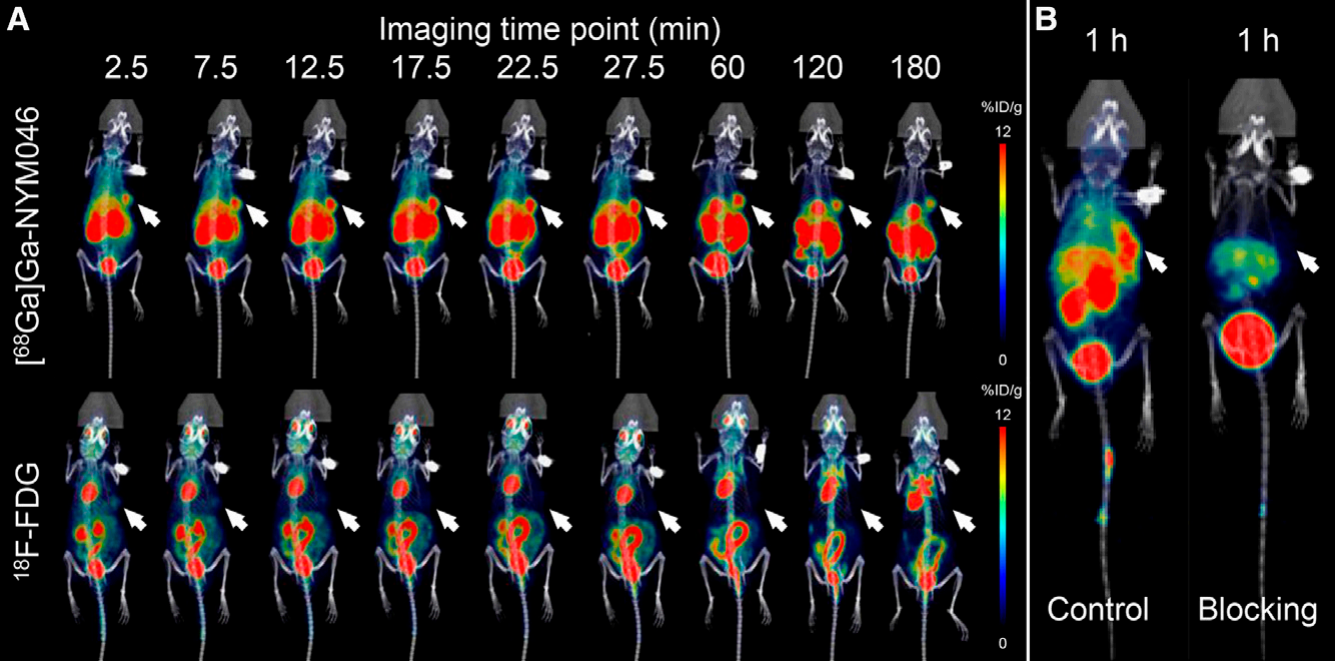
(A) In vivo biodistribution imaging of [^68^Ga]Ga-NYM046 and ^18^F-FDG in OS-RC-2 xenograft tumor models. (B) Representative PET/CT images of OS-RC-2 xenograft tumor models with or without unlabeled NYM046 blocking.
